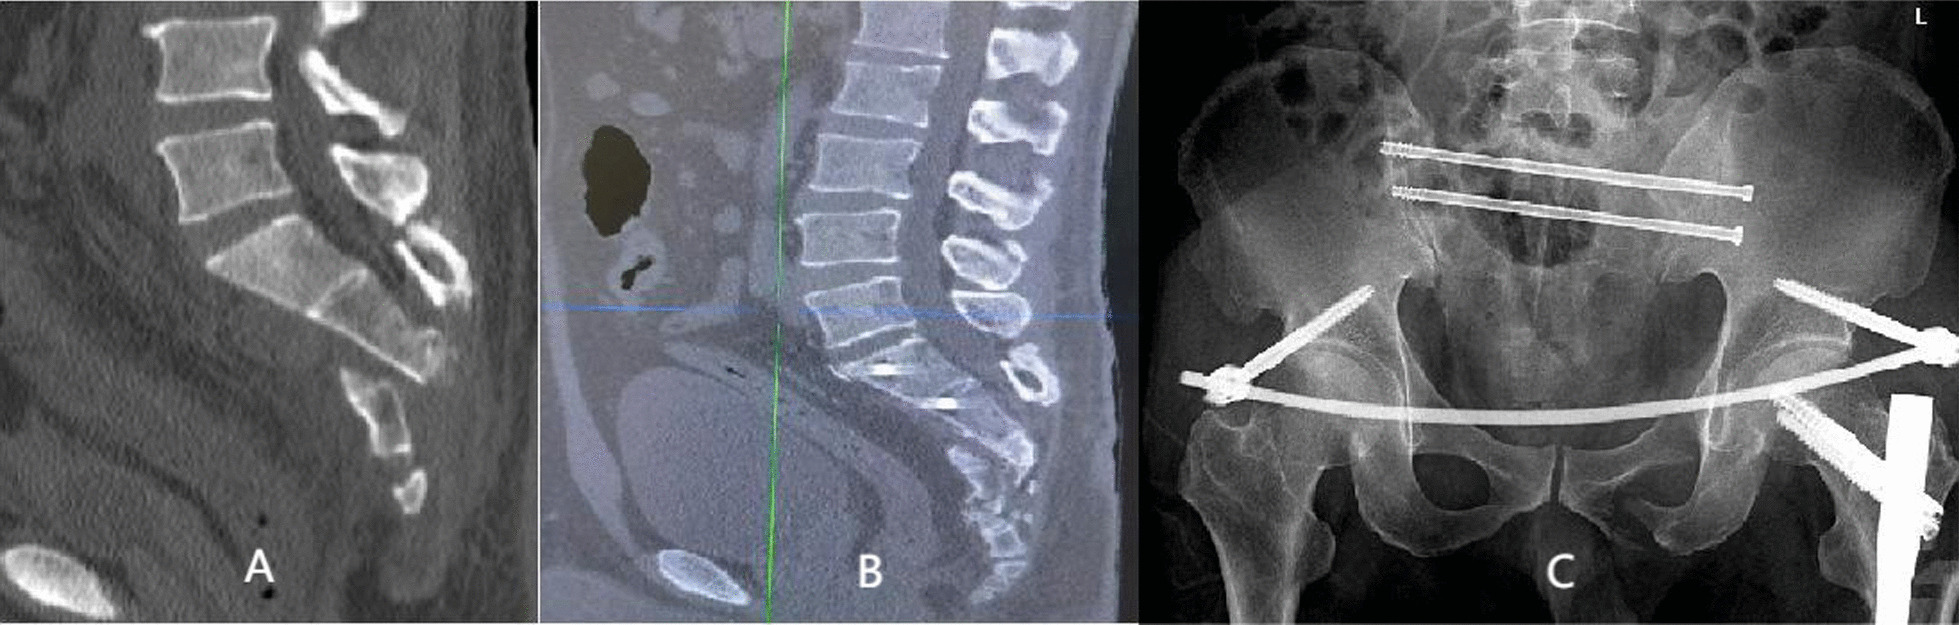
Case 2. A 44-year-old man who was injured from a fall from a height underwent S1 and S2 transiliac–transsacral screw fixation for a 301SPD type Ib fracture. A Preoperative CT scan of the sacrum in the midline sagittal plane shows kyphotic deformity. B Postoperative CT scan midline sagittal image shows unreduction of fracture. C The radiological examination image shows that the fracture was fixed satisfactorily
